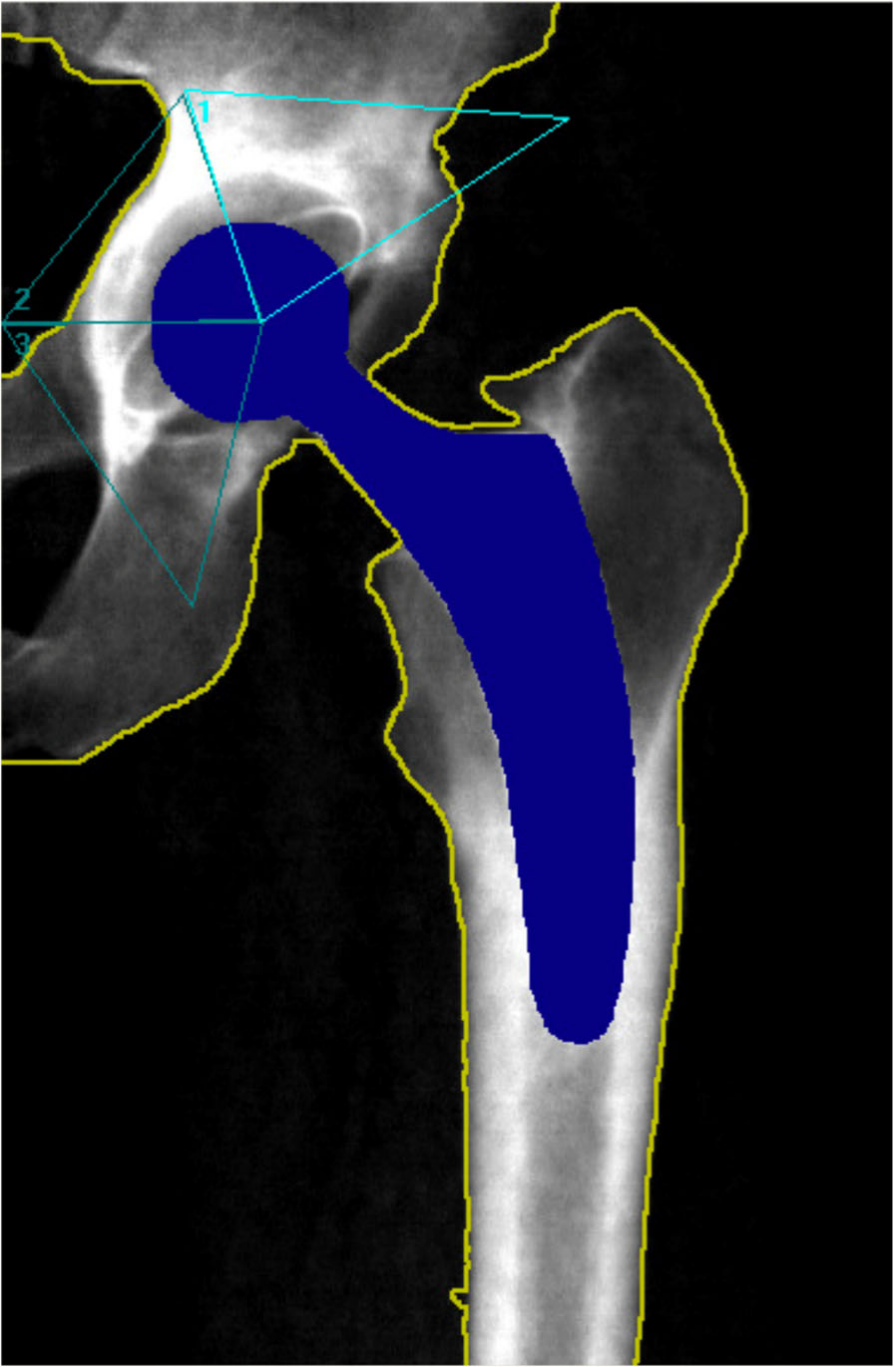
Dual-energy X-ray absorptiometry image of the prosthesis with modified DeLee and Charnley zones
